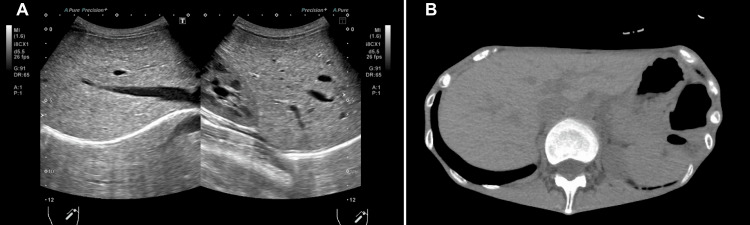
Abdominal ultrasonography and CT (A) Abdominal ultrasonography. (B) CT demonstrates no hepatic tumors, hepatomegaly, or fatty liver. CT, computed tomography.
